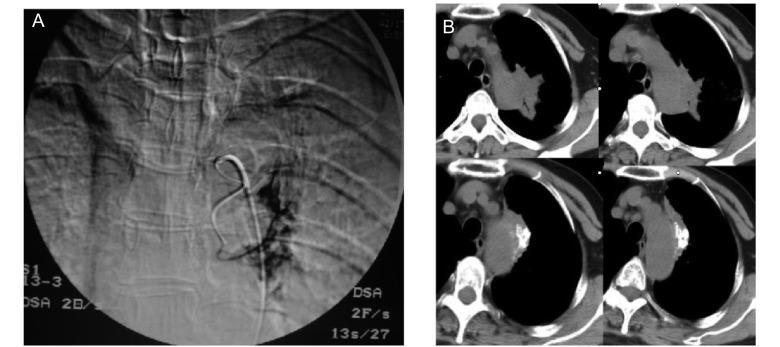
左上叶腺癌单纯碘化油乳剂栓塞化疗。A：微导管超选择插管后栓塞化疗；B（上）：肿瘤治疗前；B（下）：治疗后4个月复查肿瘤内碘化油浓聚，瘤体缩小 Adenocarcinoma of left superior lobe with simple embolization of emulsion of chemotherapeutics and iodized oil. A: Embilization and chemotherapy by superselective incubation with microcatheter; B (top): pre-treatment; B (bottom): Follow up 4 months after treatment, CT demonstrates iodized oil collection in tumor and the tumor become smaller
